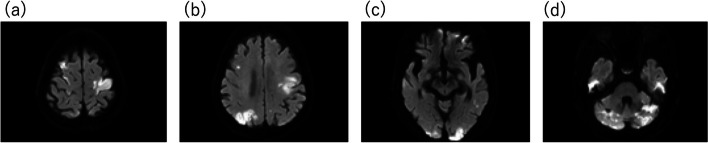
Diffusion-weighted magnetic resonance images 5 h after surgery. Multiple embolic infarcts in the bilateral frontal (a), parietal and occipital lobes (b and c), and the cerebellar hemispheres (d) were detected
